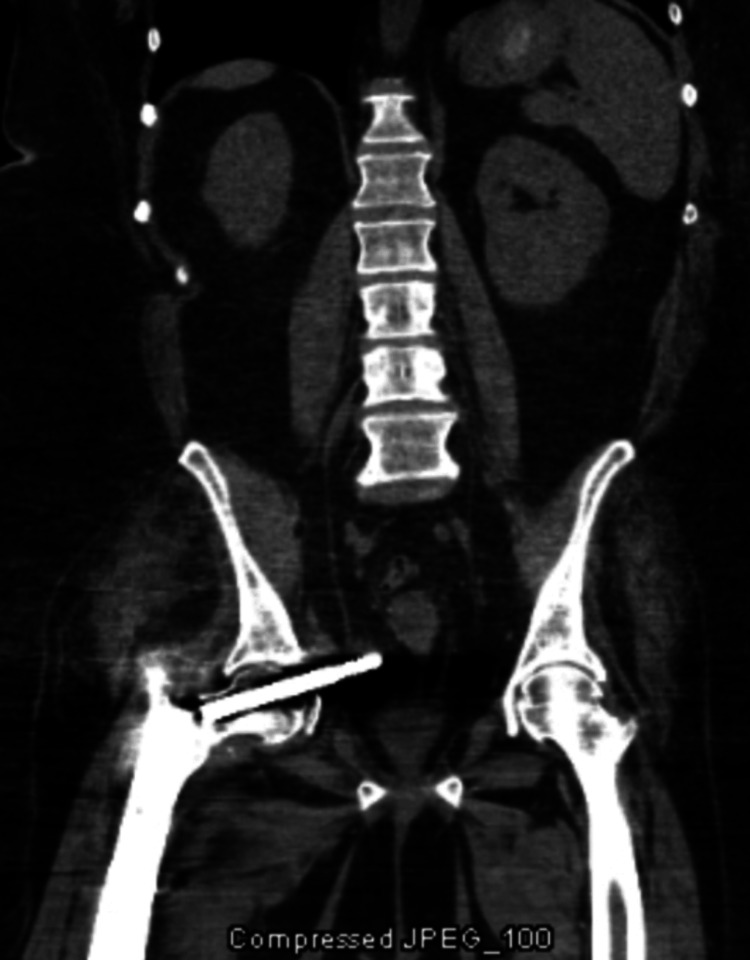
CT coronal view demonstrating intrapelvic migration of the lag screw abutting against the rectum and vagina
